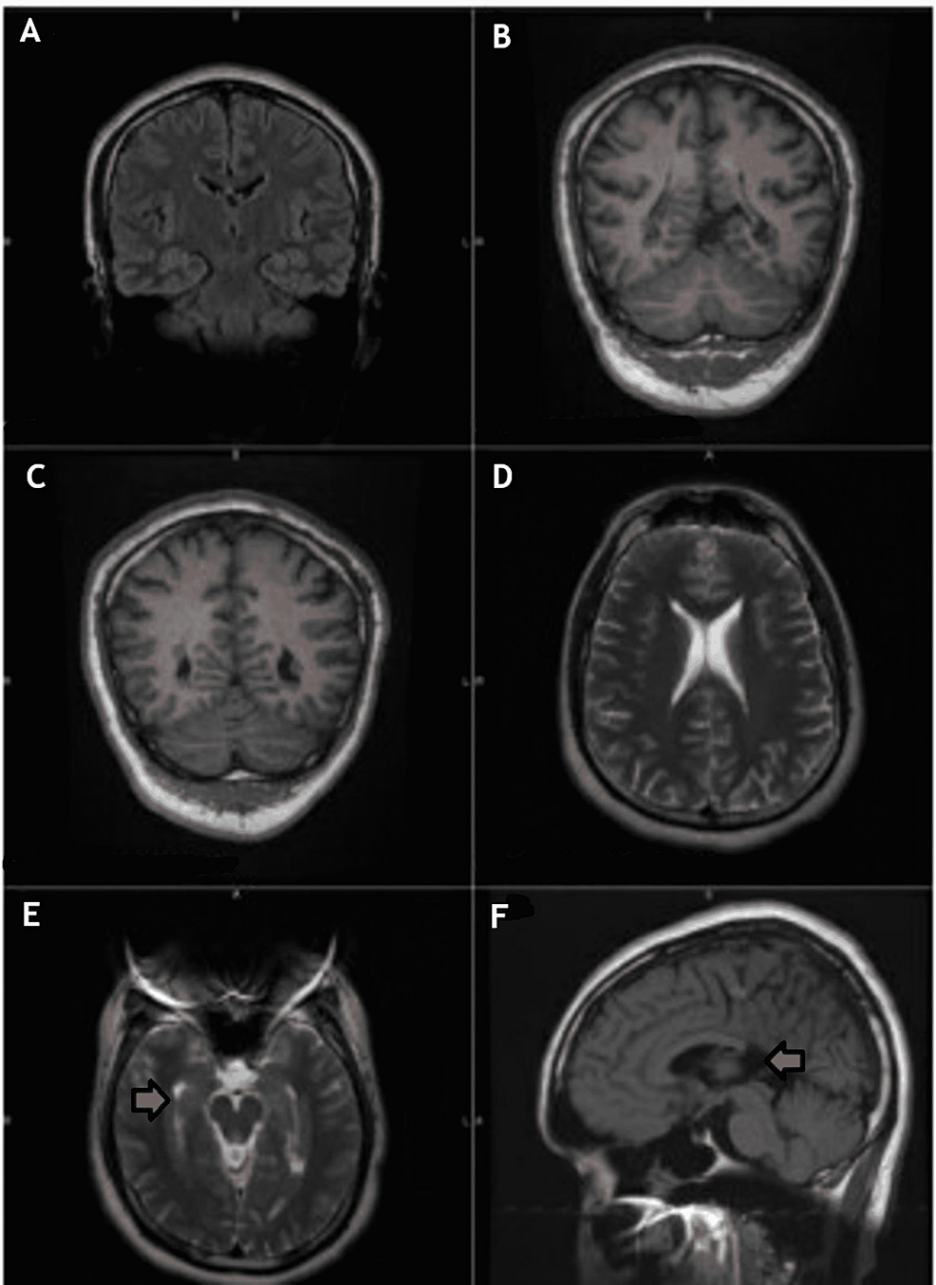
CT scan of the brain without contrast (A-F) Multiple foci of gray matter are seen along the subependymal and periventricular aspects of the temporal and occipital lobes. (E and F) The images of the brain showing development agenesis (arrows) of the splenium corpus callosum.
